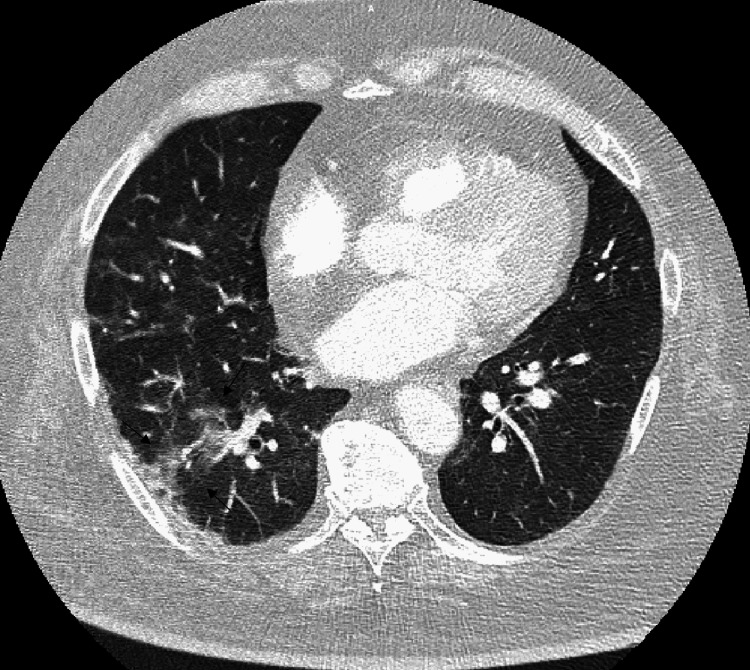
CT imaging of the lungs showing mild ground glass opacities in the right lung.
